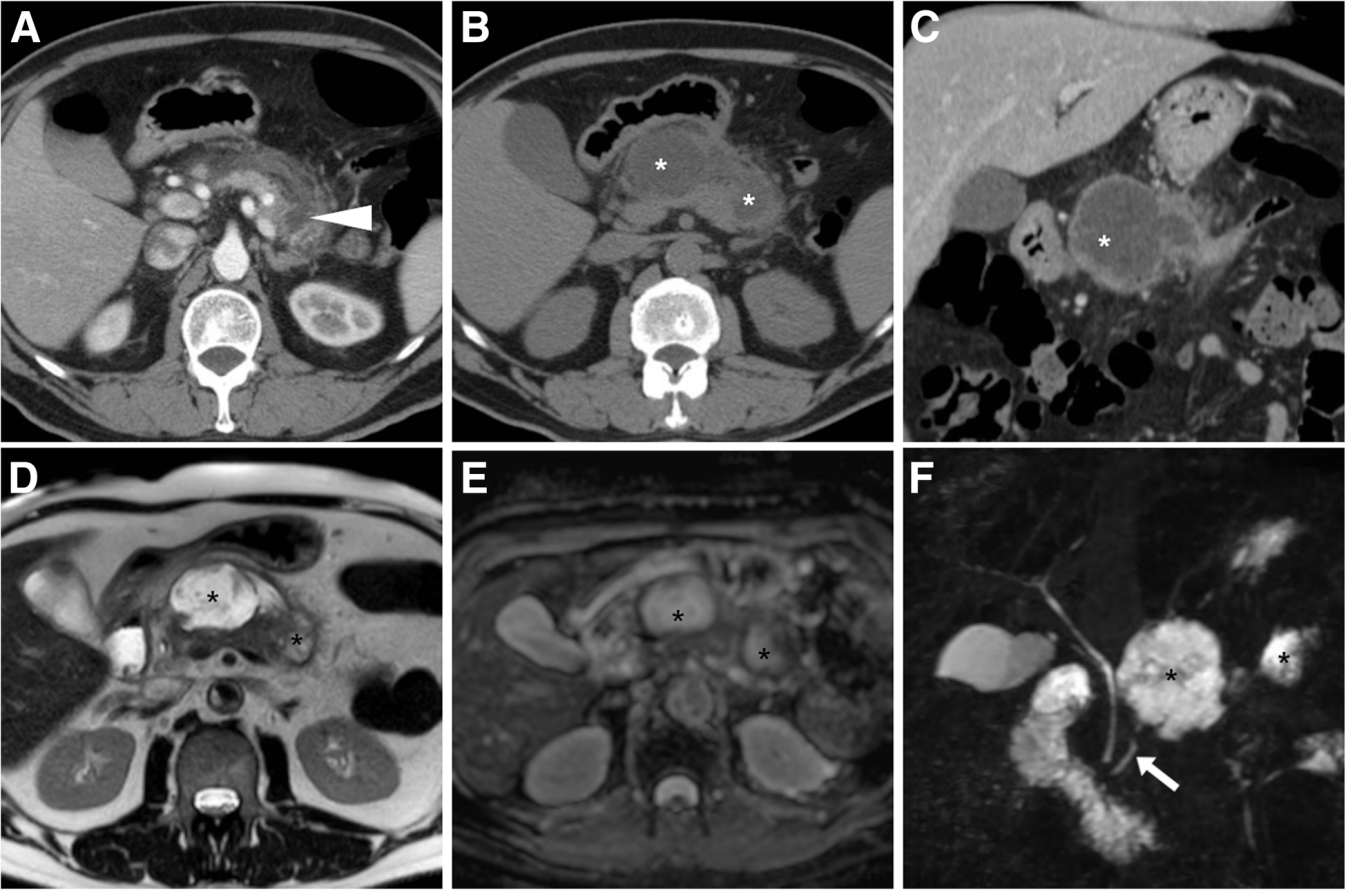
In a 55-year-old male with clinical and laboratory diagnosis of acute pancreatitis (AP), initial contrast-enhanced multidetector CT (MDCT, a) showed mild peripancreatic oedema and a limited region of nonenhancing parenchyma (arrowhead) at the pancreatic tail. Follow-up unenhanced (b) and post-contrast (c) CT showed development of a bilobated hypoattenuating collection (*) in the lesser sac. A month later, MRI confirmed diagnosis of non-infected walled-off pancreatic necrosis (WOPN) by showing persistent collection (*) with internal low-signal debris on T2-weighted images (d), without restricted diffusion on apparent diffusion coefficient (ADC) map (e). Note superimposition of collection (*) on the main pancreatic duct (MPD, arrow) on MR-cholangiopancreatography (MRCP) image (f)
